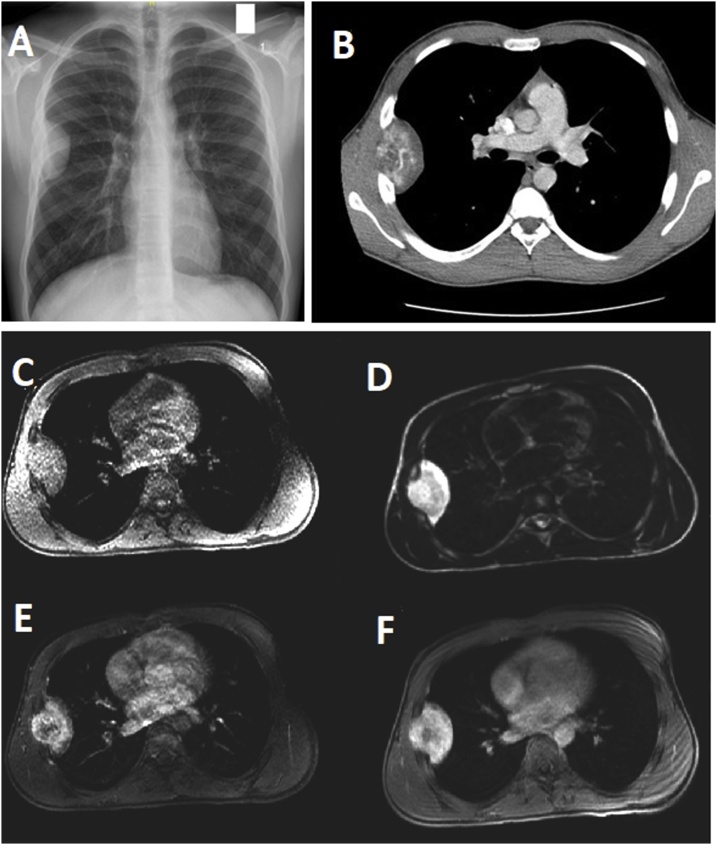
Chest X-ray with right lateral chest wall mass (A). Contrast-enhanced CT; a well-defined mass with heterogeneous enhancement arising in the 5th interspace (B). Tl-weighted image shows a solid mass with a low signal intensity but a little higher compared to that of the adjacent muscles in the right chest wall (C). The fat-suppressed T2-weighted image reveals homogeneous mass with high signal intensity similar to that of fat tissue, which was of high-intensity on fat-suppressed MRI (D). Early phases after administration of contrast, a characteristic heterogeneous enhancement of the tumor (E) that became even more uniform during the delayed phase (F).
